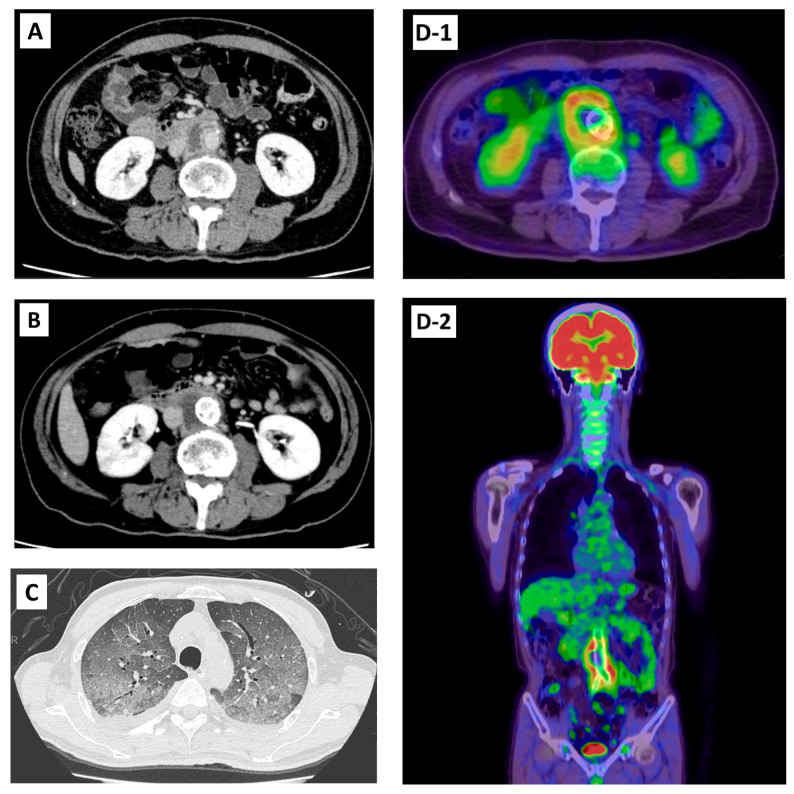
Radiological findings of the abdominal aortic aneurysm and lung involvement. (A) Contrast-enhanced computed tomography (CT) in April 2024 showing an abdominal aortic aneurysm without obvious periaortic soft tissue enhancement; (B) Contrast-enhanced CT in August 2024 demonstrating rapid enlargement of the abdominal aortic aneurysm with increased enhancement and thickening of the surrounding soft tissue; (C) Chest CT in August 2024 showing diffuse bilateral ground-glass opacities (GGOs) in the lungs. (D) ^18^F-fluorodeoxyglucose positron emission tomography/CT (FDG-PET/CT) performed in August 2024 demonstrating intense FDG uptake along the aneurysmal aortic wall. (D-1) Axial (transverse) fused FDG-PET/CT image showing intense FDG uptake along the aneurysmal aortic wall. (D-2) Coronal fused FDG-PET/CT image likewise showing intense FDG uptake along the aneurysmal aortic wall.
